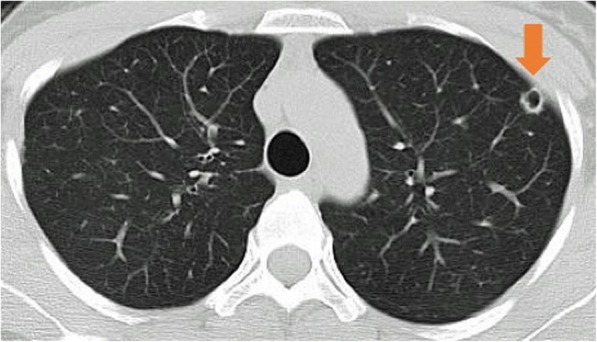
Metastasis findings of case 4. CT image shows multiple pulmonary metastases
